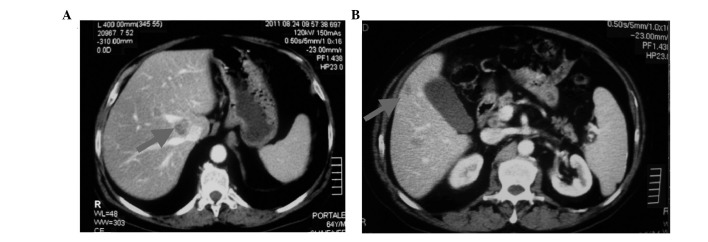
Computerised tomography (CT) scan performed on December 10th 2011, demonstrating a partial response of liver metastases with maximum diameters of 1.7 cm (A) and 1 cm (B).
